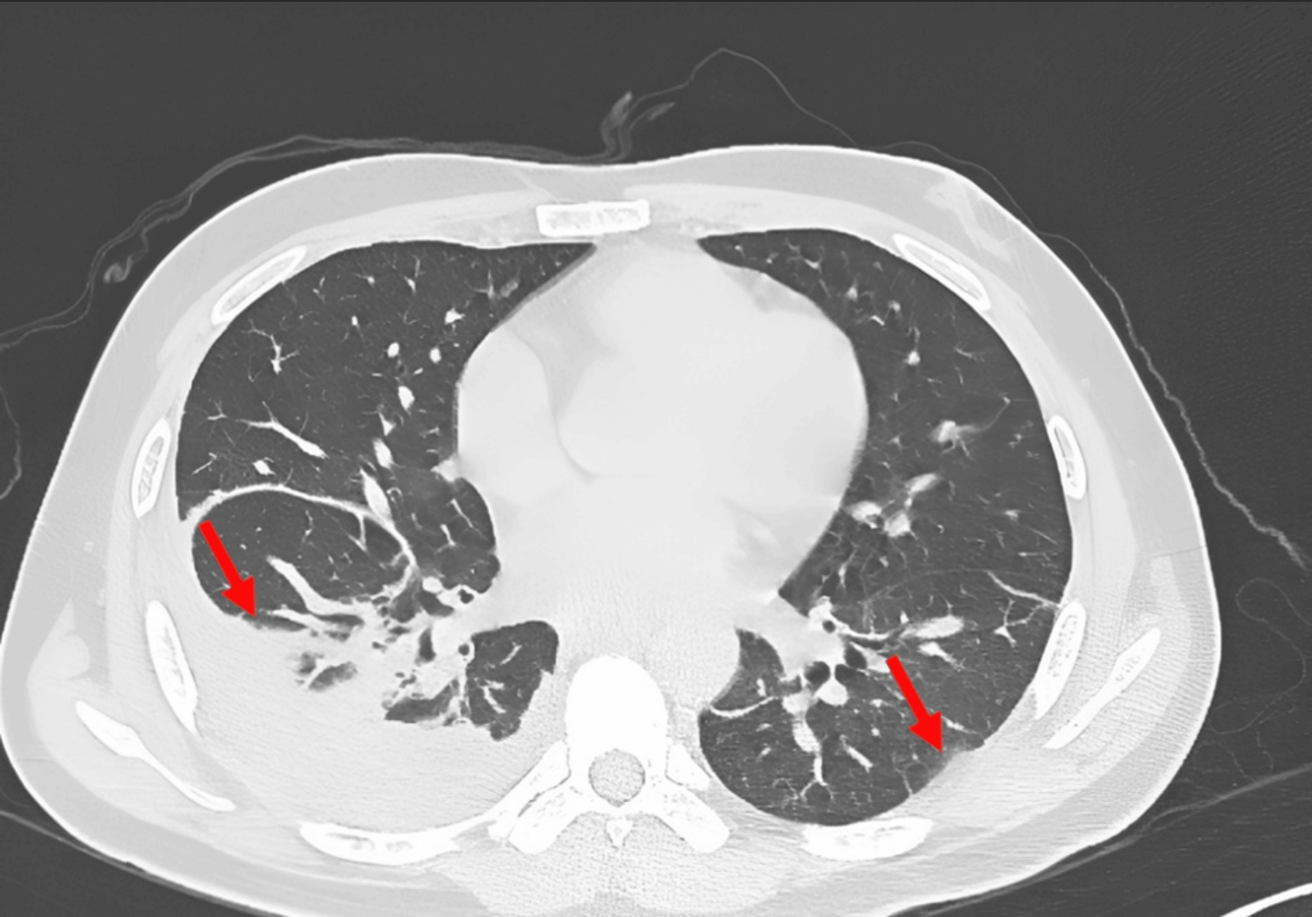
Follow-up CT showing regressive course of the disease mainly on the left side (red arrows)
